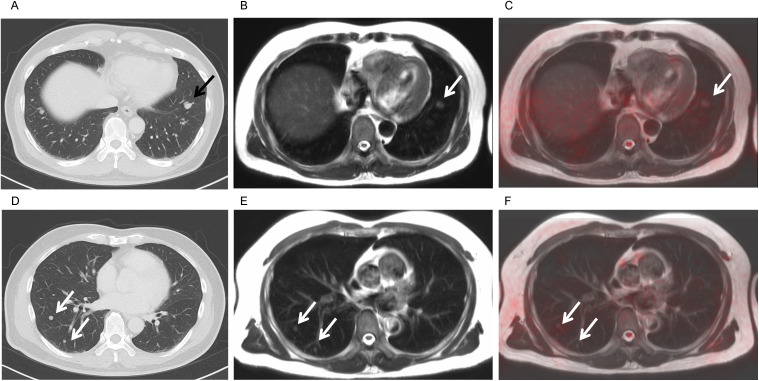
Comparison of lung metastasis detection between computed tomography (CT) and magnetic resonance imaging (MRI). Images A–C and D–F correspond to the same metastatic lung lesions. A and D: Axial chest CT clearly demonstrates lung metastases. B and E: Corresponding T2-weighted MRI shows lesions with reduced conspicuity. C and F: Fusion images of T2-weighted and diffusion-weighted imaging (DWI) failed to visualize the lesions, indicating the limited sensitivity of DWI in detecting small lung metastases.
